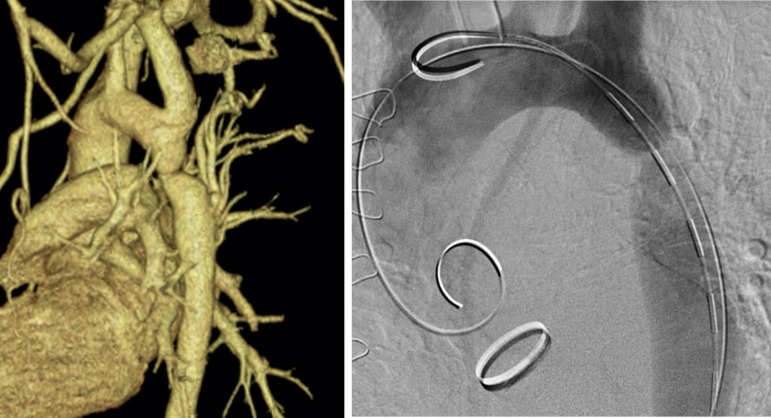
A) Preprocedural computed tomography angiography revealing coarctation of the aorta just at the origin of the left subclavian artery; B) Intraprocedural angiography confirming the previous findings.
